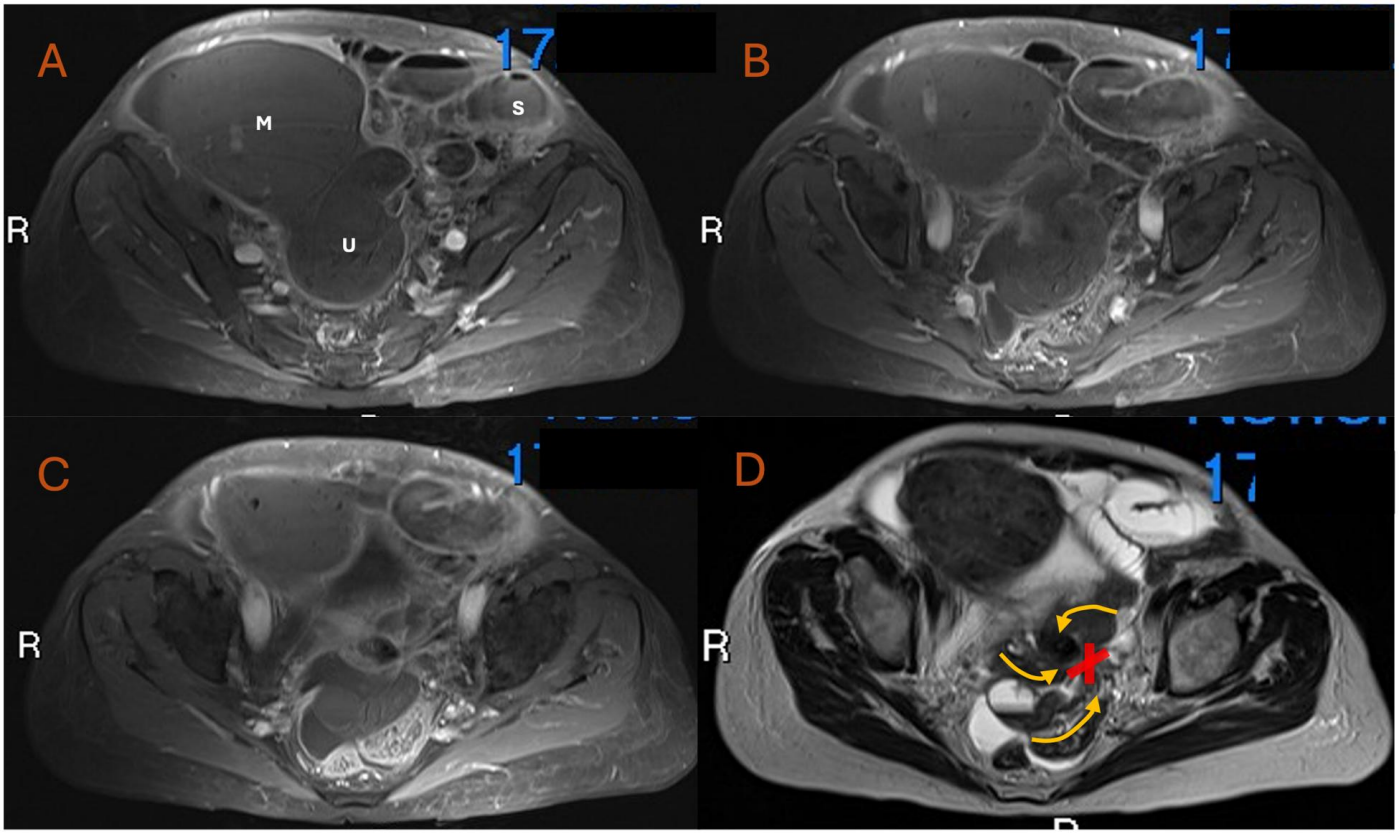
Day 12 of admission MRI pelvis with contrast—axial slices. (A-C) *Post-contrast axial T1-weighted fat suppressed images* moving caudally with the uterine fibroid mass (M) and uterus (U) identified. The key finding is the total lack of contrast uptake within the uterus, adnexal structures and associated fibroid mass highly suggestive of a local ischaemic cause. Ongoing mild small bowel upstream dilatation noted (S). The background “whirlpool sign” is again demonstrated of the un-enhancing uterine cavity. (D) *Annotated non-contrast T2-weighted axial image* of the same slice as C depicts the “X sign” which is a central focal point of tethering to the left of the uterine cervix/vaginal wall where the uterus and adnexal structures appear to wrap around. The wrapping adnexal structures around the “X” are labelled with corresponding curved arrows. The “X-sign” is also demonstrated on the contemporaneous contrast sequence of the same slice (C). Abbreviations: M = Uterine mass, suspected large fibroid; X = “X sign”; S = small bowel; U = Uterus; Curved arrowhead = direction of travel of uterine and adnexal structures.
